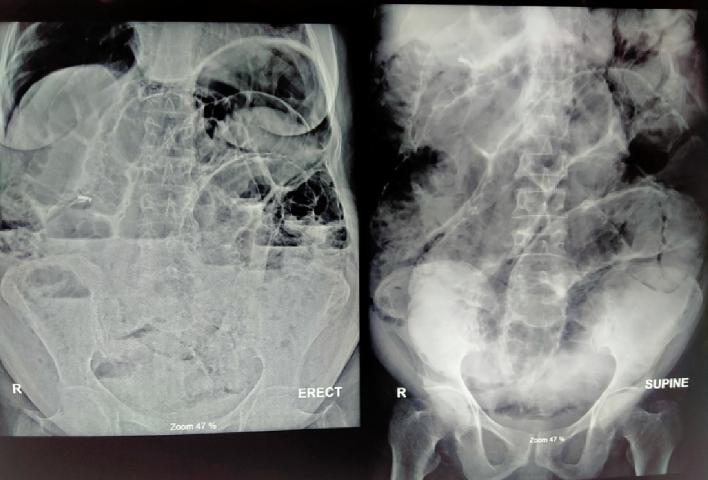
Showed multiple air fluid level in erect and distended bowel in supine abdominal X-ray.
